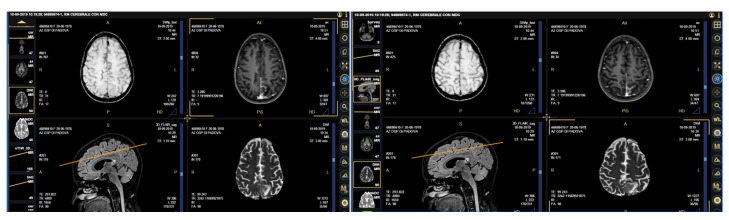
Cerebral MRI (Magnetic Resonance Imaging) highlighting the presence of leptomeningeal metastases at the bottom of the right central sulcus, on the surface of the convolutions of the left pre-wedge, with associated edema of the underlying parenchyma.
